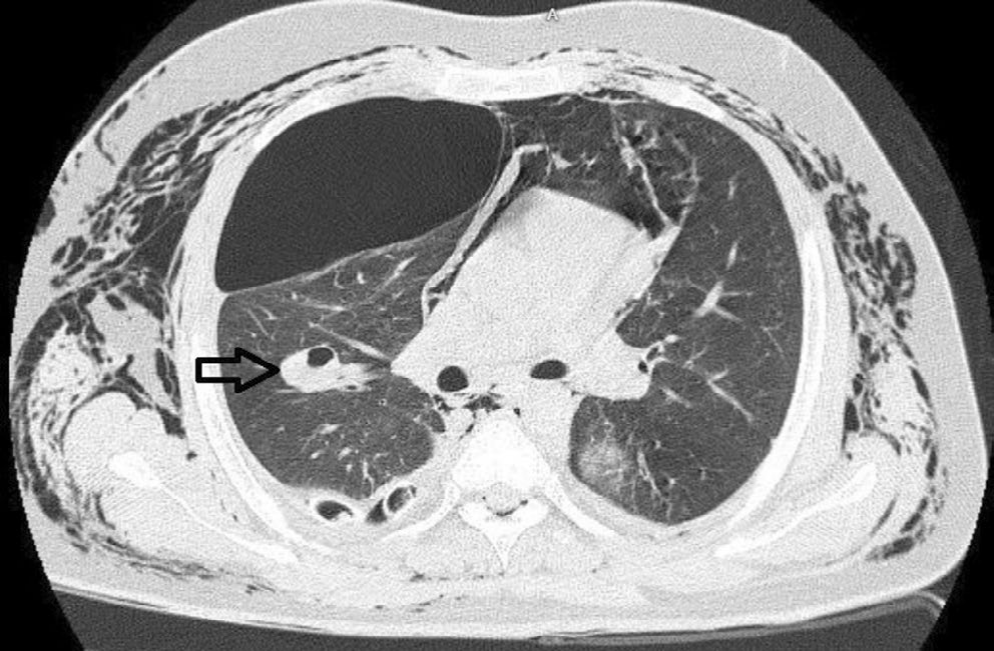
A ruptured hydatid cyst in lung CT scan (hollow arrow).
